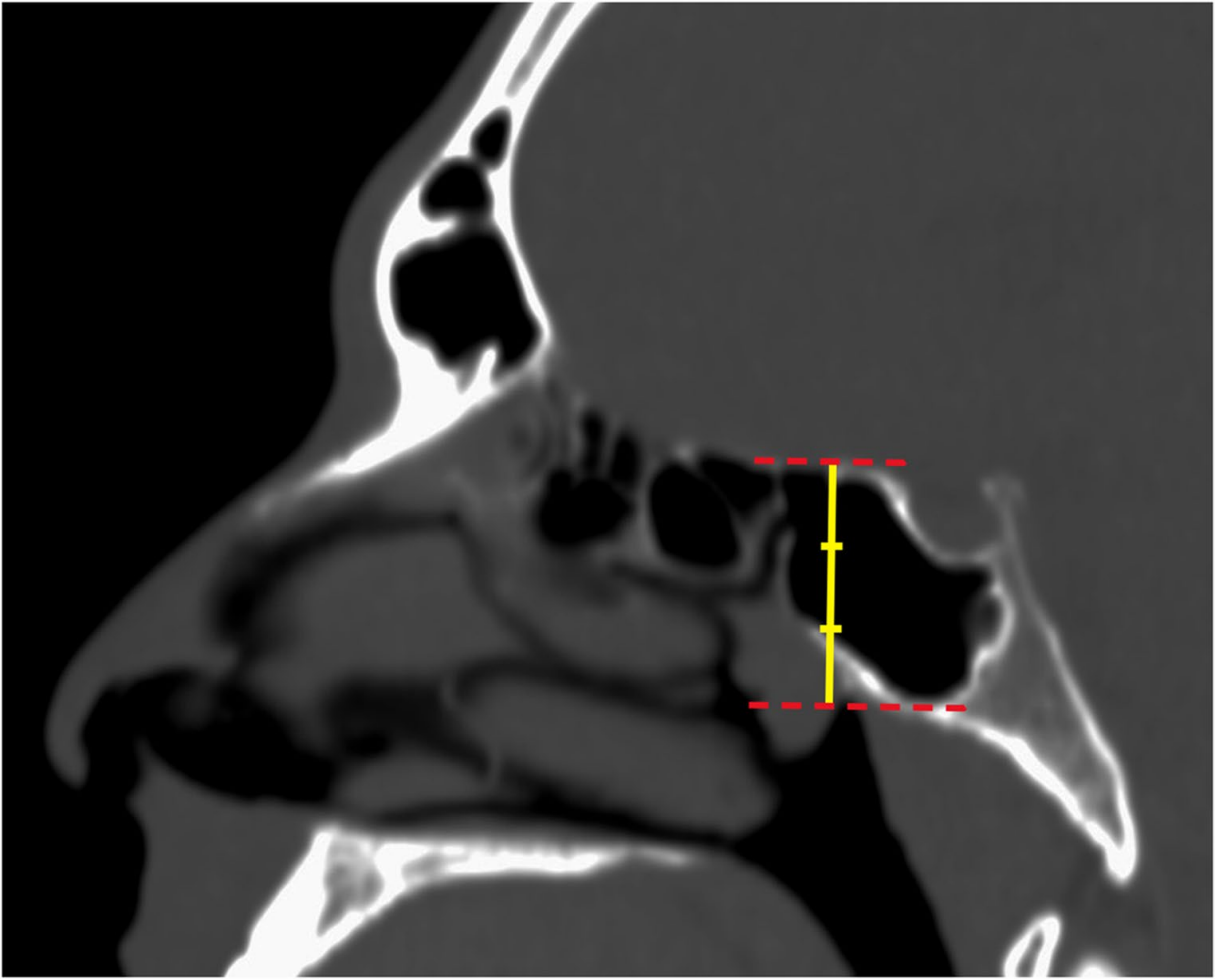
Sagittal CT image demonstrating the vertical reference line divided into three equal segments to determine the vertical position of the sphenoid sinus ostium. Red dotted lines represent the superior and inferior boundaries of the sphenoid sinus, while the yellow line indicates the vertical reference line
